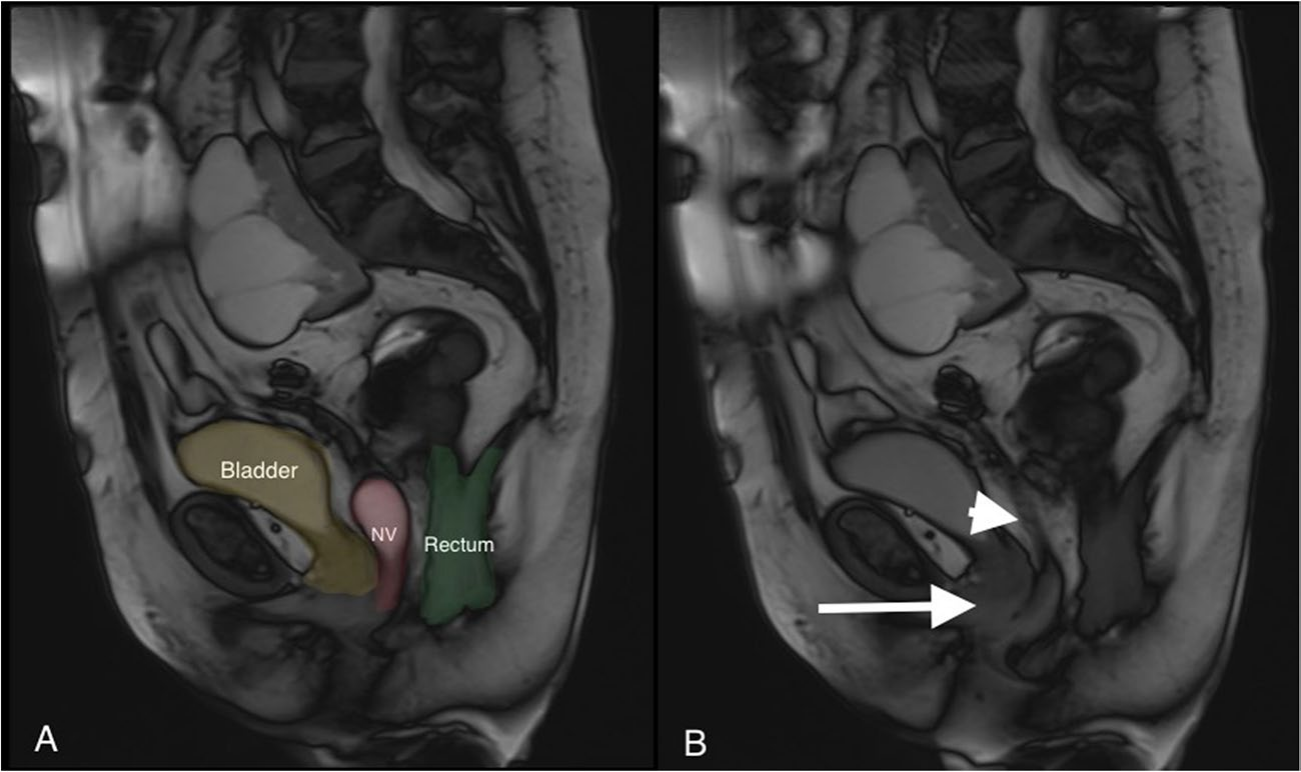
Dynamic pelvic MRI. A At rest, B at maximum Valsalva full-thickness prolapse of the mucosa of the neovagina (NV) with intussusception shown (*white arrow*) with the remaining neovagina staying in place (*head arrow*)
